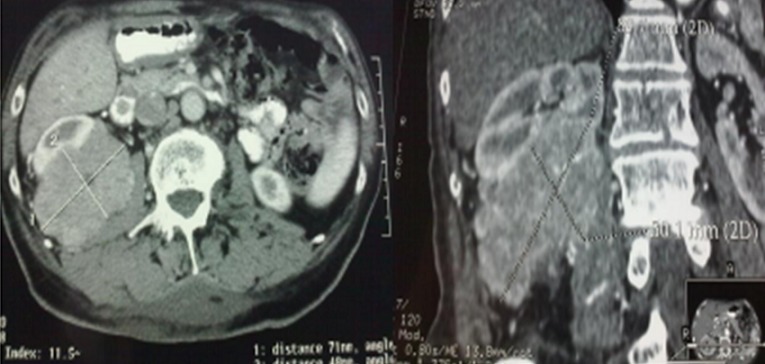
Abdominal CT scan showing the retroperitoneal localization
